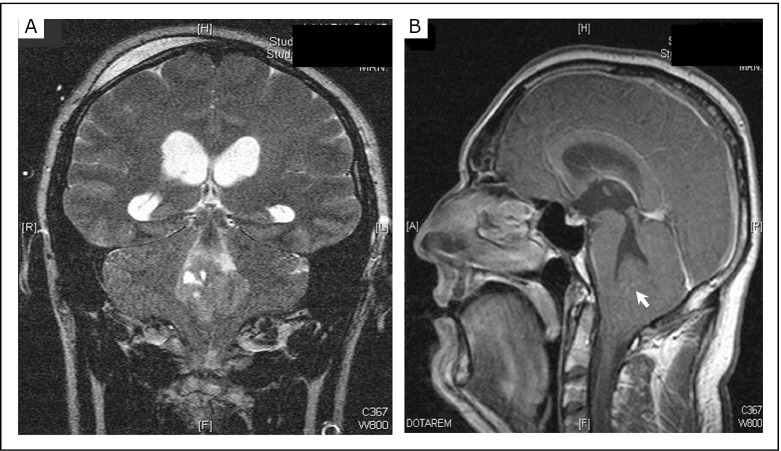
Coronal T2-weighted MRI (A) demonstrating a lesion in the fourth ventricle resulting in an outflow obstruction of the CSF at the foramina of Luschka and Magendie, and a dilatation of the third and fourth ventricles and the temporal horns of the lateral ventricles. Sagittal T1-weighted image with gadolinium enhancement (B) revealing the lesion with minimal contrast enhancement leading to a cranial displacement of the inferior medullary velum (arrow) and a cerebellar tonsillar herniation with nearly total obstruction of foramen magnum.
